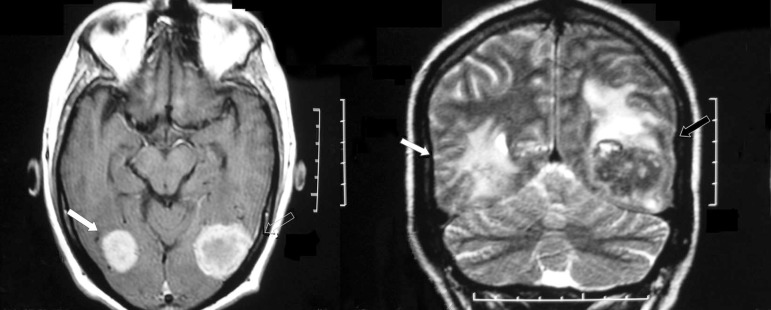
Preoperative MRI: arrows showing bilateral occipito-temporal metastasis
